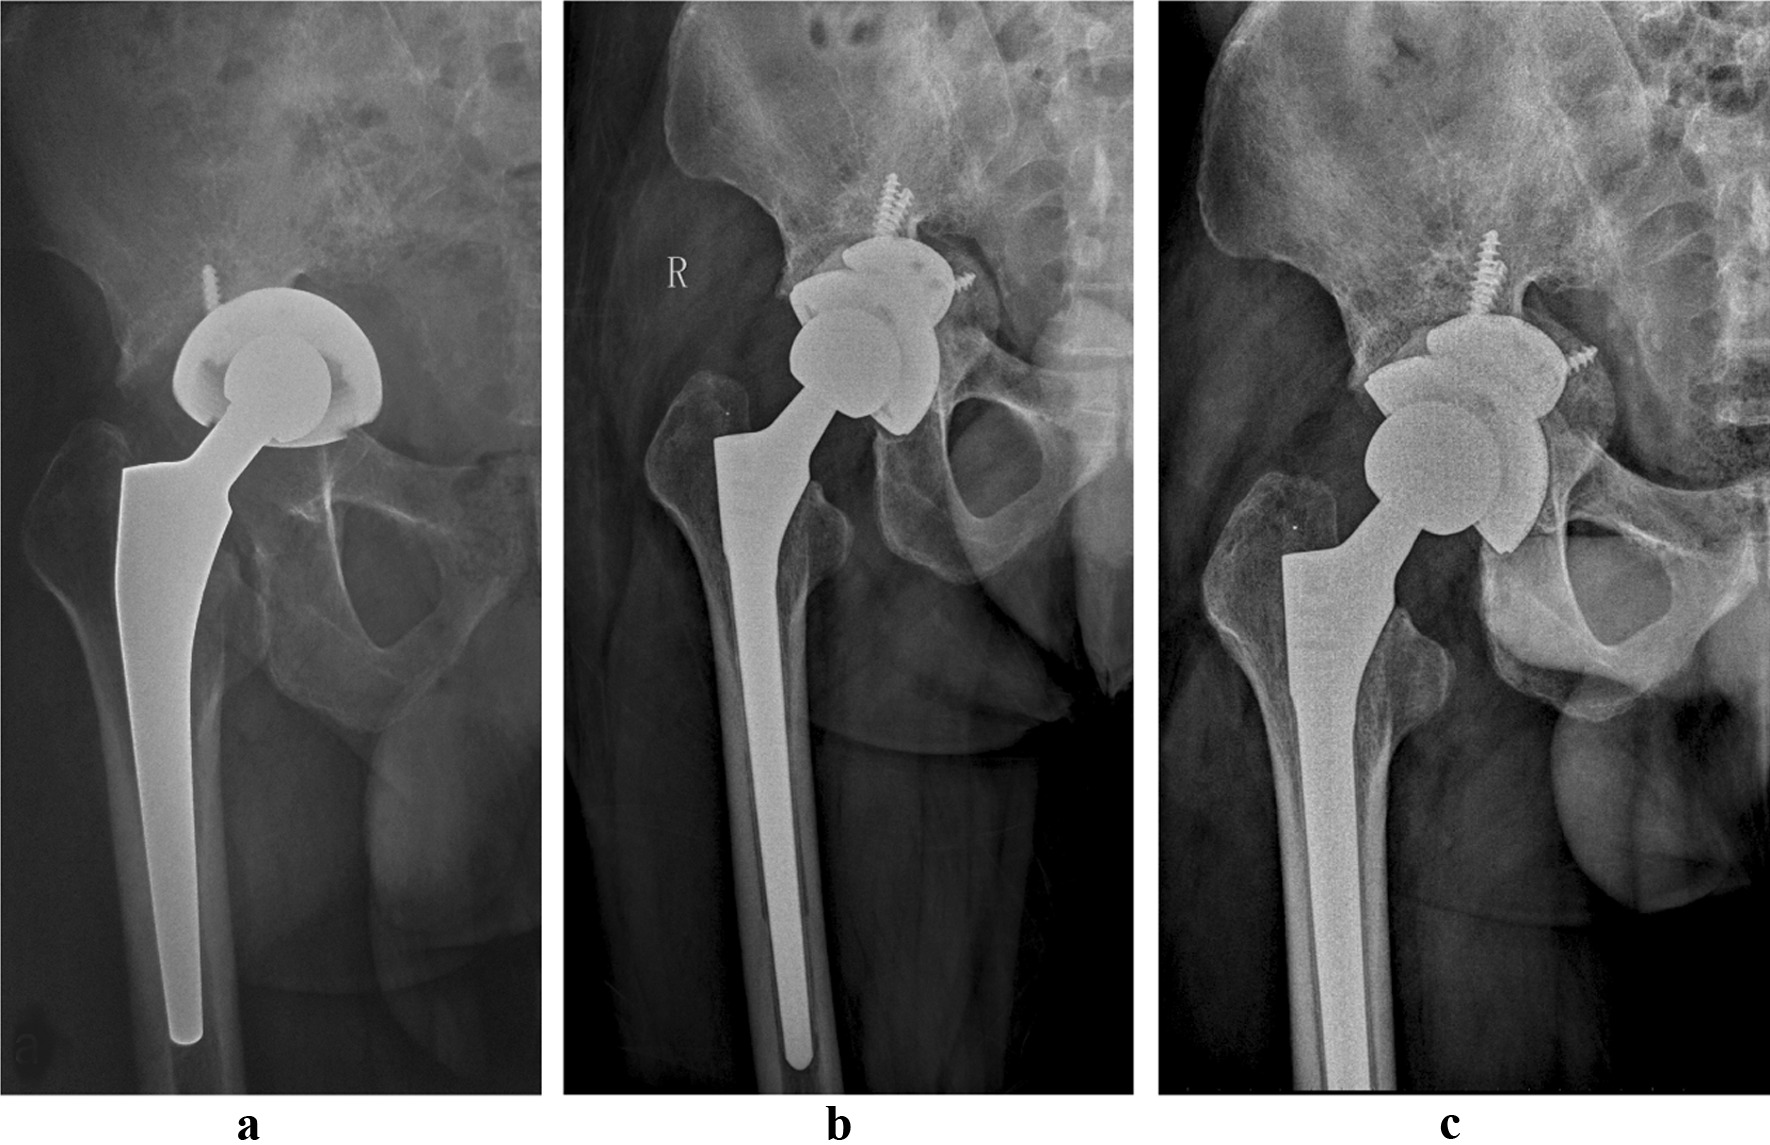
AP radiograph of a 57-year-old male patient with a type IIC bone defect revised for aseptic loosening. a before surgery; b three days after revision surgery; c X-ray with radiolucent lines in the three zones and aseptic loosening of the cup at 36 months postoperatively
